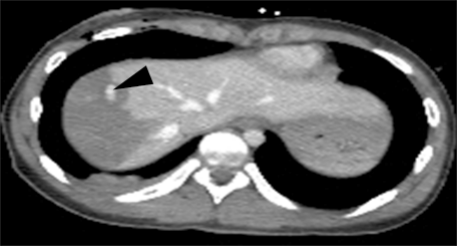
Abdominal CT. Degree Ⅳ liver laceration. Black arrow – segment Ⅷ metallic fragment
